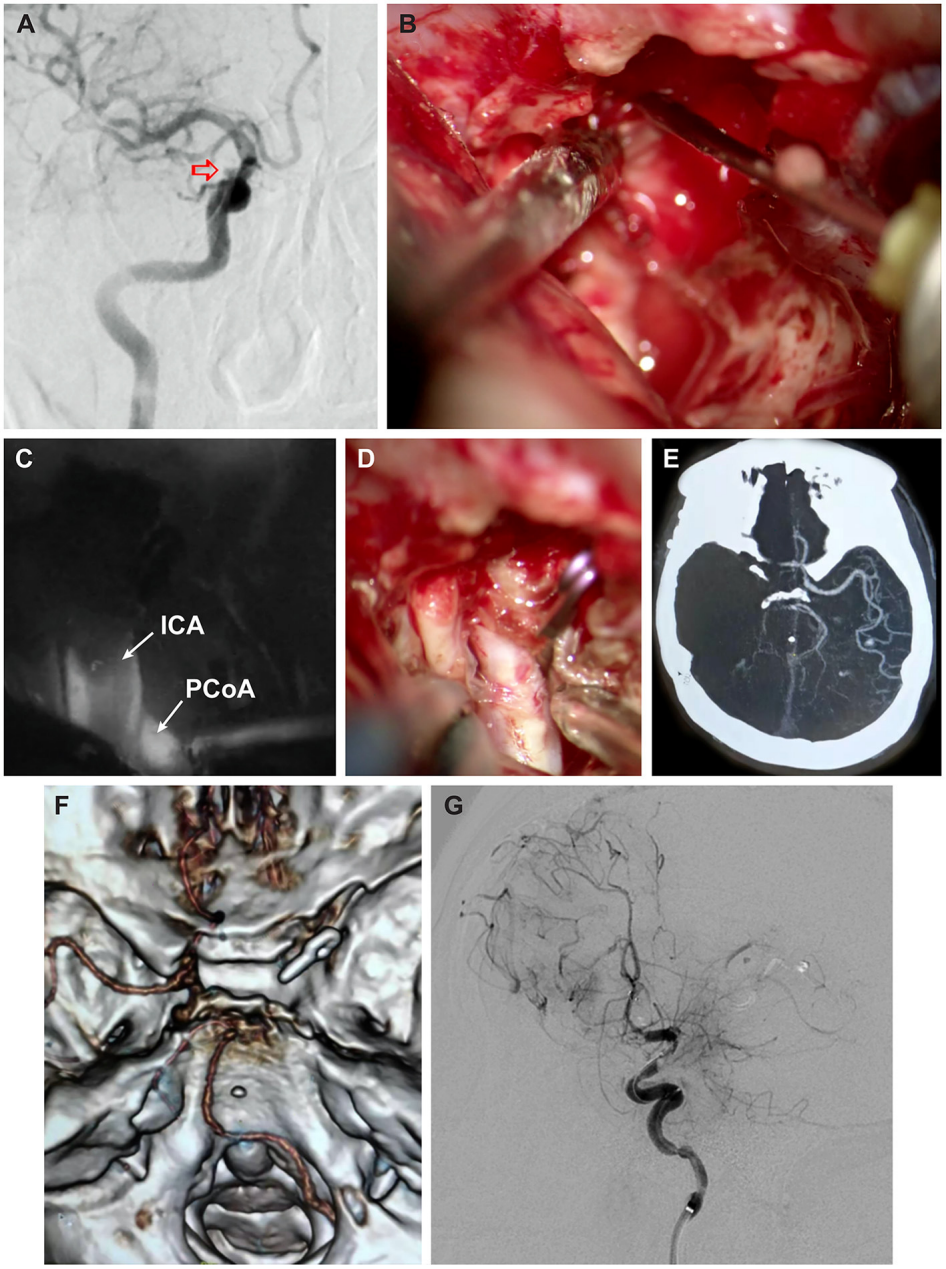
Case 1. (A) Digital subtraction angiography (DSA) oblique view demonstrating small unruptured aneurysm (*red arrow*) in the transition between the cavernous and clinoidal segments of the right internal carotid artery (ICA). (B) Intraoperative photograph of direct injection of fibrin glue into the cavernous sinus posterior to the ICA. (C) Indocyanine green (ICG) videoangiography demonstrating patency of the right ICA, darkened by the presence of atheroma in the center of the vessel, and patency of the right posterior communicating artery (PCoA) and (D) companion intraoperative photograph. (E) Postoperative axial computed tomography angiography (CTA) and (F) 3-dimensional CTA, superior skull base view, obtained 2 h after surgery, demonstrating complete occlusion of the right ICA. (G) Postoperative oblique-lateral DSA after several thrombectomies showing patency of the right ICA and contrast stagnation caused by poor collateral flow. Used with permission from Henan Provincial People's Hospital, Juha Hernesniemi International Center for Neurosurgery.
